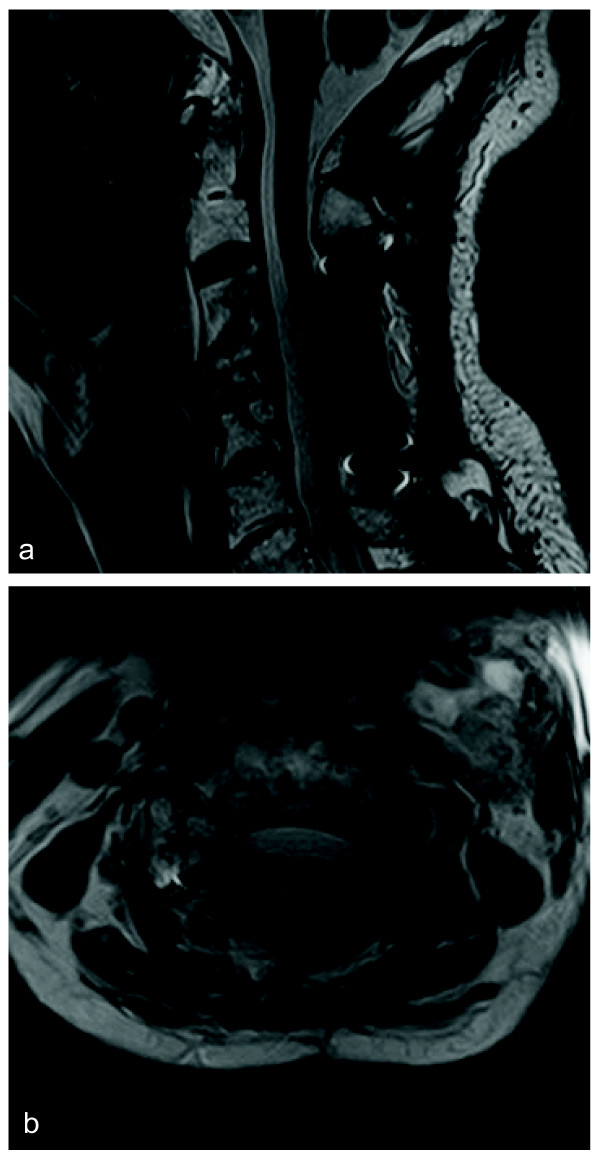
Postoperative sagittal (a) and axial (b) T2-weighted magnetic resonance image showing a reduced tumor size and spinal cord decompression.
